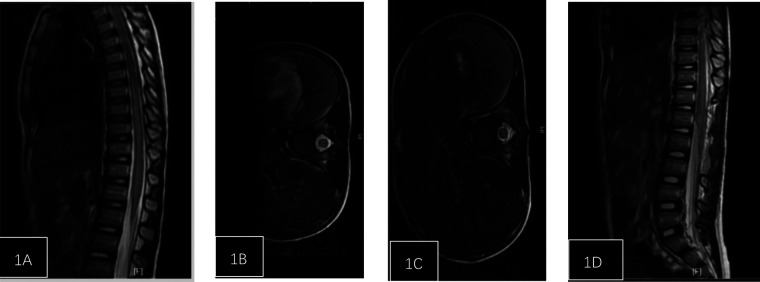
Patient, female, 5 years and 1 month, injured from a fall. (A) Post-injury spinal MRI shows edema of the spinal cord from T6 to L2 and dilatation of the central canal. (B) Axial T2-weighted imaging shows high signal intensity. (C) One week post-surgery follow-up spinal MRI shows reduced edema compared to pre-treatment, with no central canal dilation observed. (D) Axial T2-weighted imaging.
